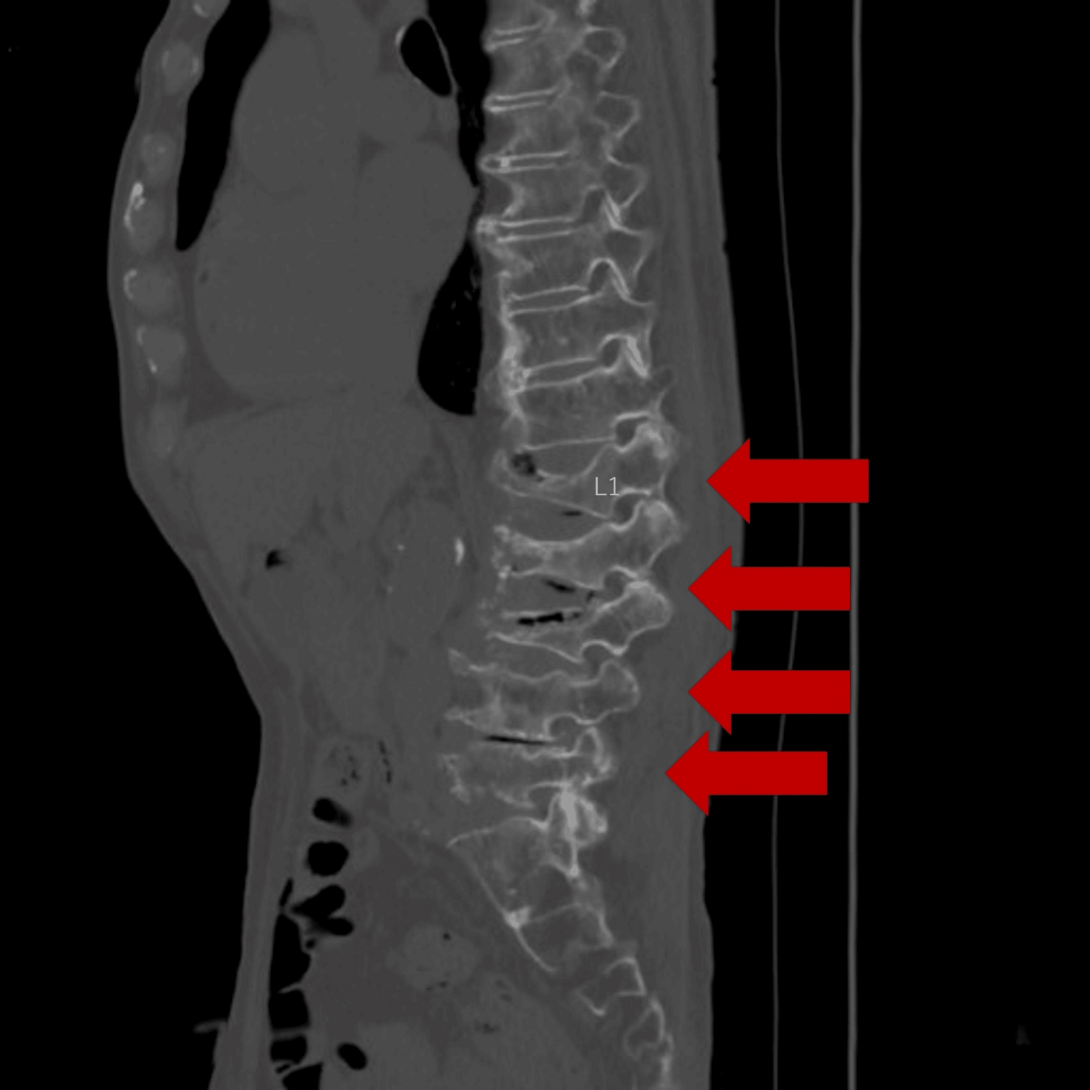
Lumbar spine CT Compression fractures were identified from Th12 to L5 (arrows). CT: computed tomography
